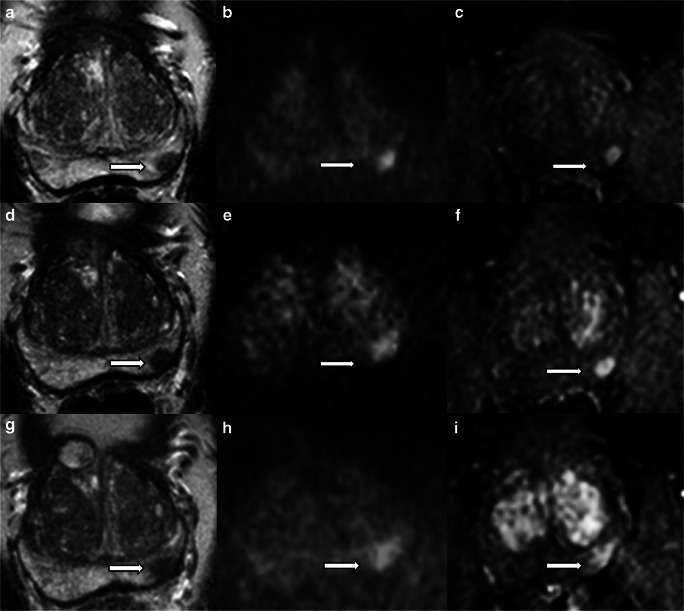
Fifty-year-old man on active surveillance for Gleason 3 + 3 (4 mm) prostate cancer in the left peripheral zone on standard transrectal ultrasound biopsy. The mpMRI scan at baseline (a, b, c) shows a left-sided peripheral zone lesion (arrows) between 4 and 5 o'clock characterised by low signal intensity on T2-weighted imaging (a), restricted diffusion on diffusion-weighted imaging (b) and early enhancement on dynamic contrast-enhanced imaging (c). The lesion showed progressive radiological change in volume and conspicuity after one (d, e, f) and two (g, h, i) years (PRECISE score: 4). The patient was treated with high-intensity focused ultrasound
